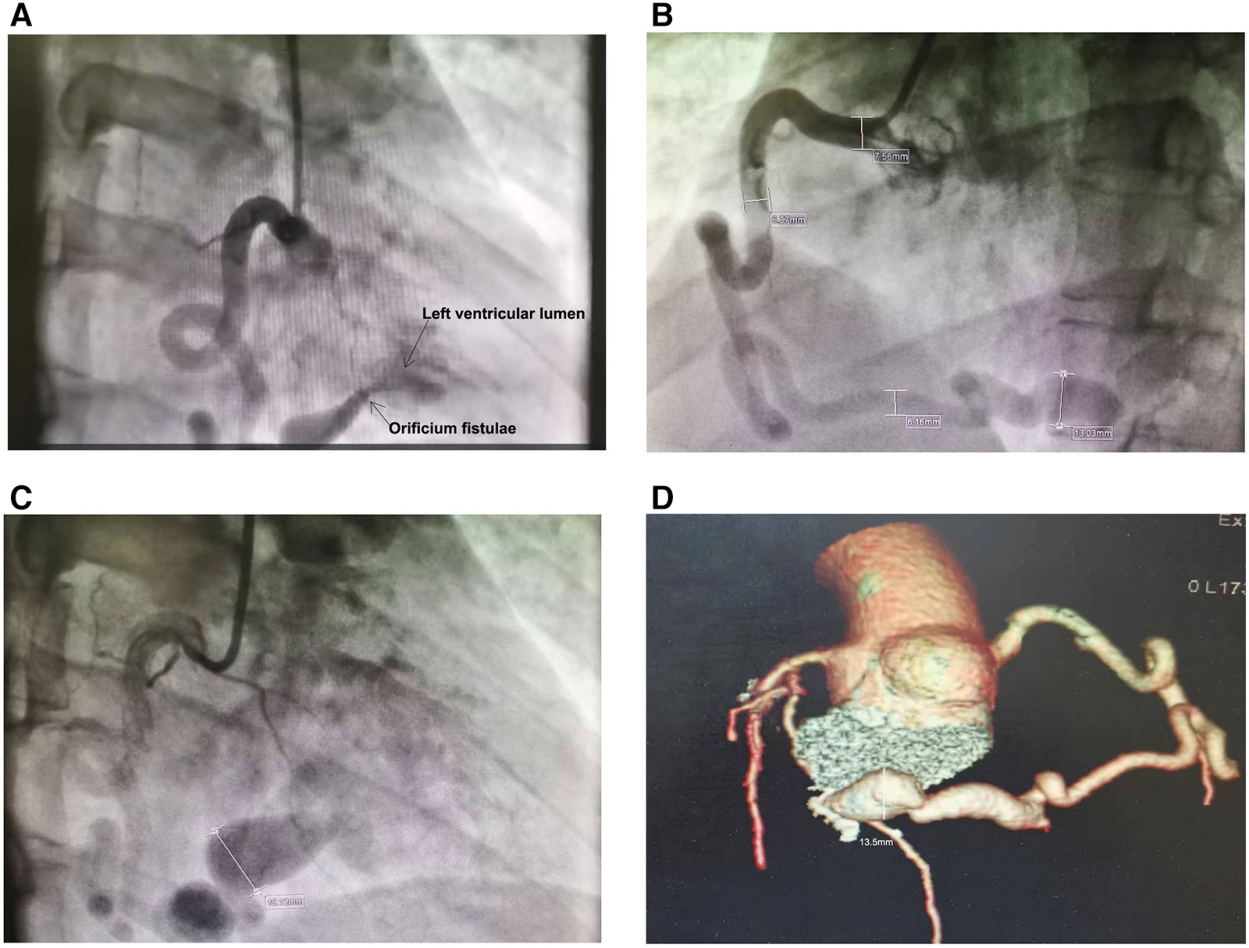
Right coronary artery to left ventricular fistula complicated with coronary artery dilation. The contrast agent flowed directly into the left ventricular lumen through the right coronary artery fistula, and the arrows point to the left ventricular lumen and orificium fistulae respectively (A) The lumen diameter of the right coronary artery is 7.56 mm in the proximal part (B), 6.37 mm in the middle part (B) and 19.12 mm in the distal part (C). In the sixth year after surgery, Coronary artery computed tomography indicated that the diameter of the distal coronary artery aneurysm in the patient's RCA was 13.5 mm (D).
